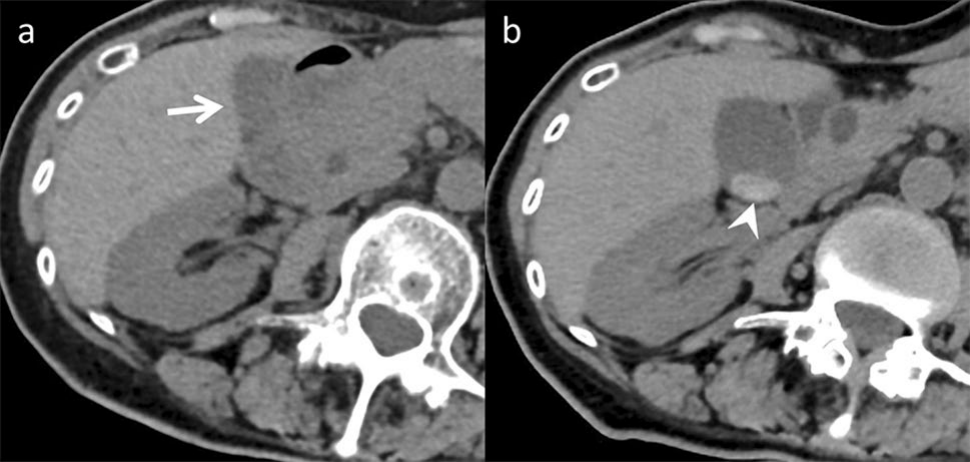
Ceftriaxone (CTRX)-associated gallbladder pseudolithiasis in an asymptomatic 65-year-old woman with diabetes mellitus, who was undergoing antibiotic therapy for iliopsoas abscesses, including CTRX. a Unenhanced computed tomography (CT) demonstrates no gallbladder abnormalities before CTRX initiation (arrow). b A high-attenuation object (stone pattern) is observed in the gallbladder on unenhanced CT performed two weeks after CTRXinitiation (arrowhead). No examination using contrast material between the CT examinations was performed. It is difficult to differentiate pseudolithiasis from gallbladder stones on a single image alone
